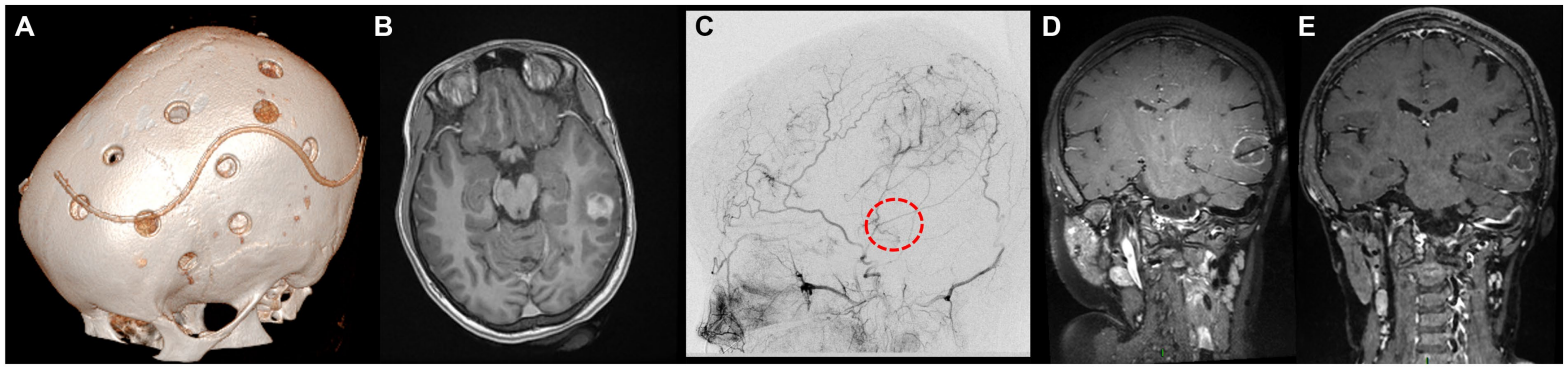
Panel showcasing the most relevant events in the medical history of L.M. (A) Day 1 postoperative cranial CT scan showing the position of the burr holes at the left side after revascularization surgery (9 years old). (B) Axial enhanced T1-weighted cerebral MRI at the age of 11, showing the appearance of a left middle temporal gyrus lesion, nodular with a small posterior cyst, surrounded by brain edema. (C) DSA of the branches of the external carotid artery showing the revascularization of the left hemisphere by the middle meningeal artery and posterior auricular artery. The red circle represents the putative location of the tumor. (D) Coronal enhanced T1-weighted cerebral MRI showing the trajectory of the LITT fiber at the end of the procedure. (E) Coronal enhanced T1-weighted cerebral MRI 10 months after the surgery, documenting the reduction in tumor size.
